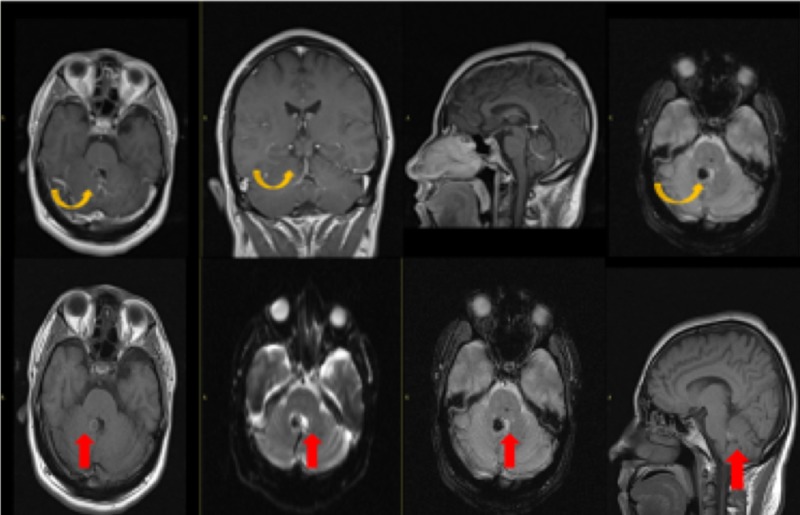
Magnetic Resonance Imaging with Contrast Post-contrast images demonstrate a focal area of blooming at the previously seen site of high density on CT at the right medial margin of the fourth ventricle without enhancement on post-contrast (yellow arrow) representing cavernoma. There is also demonstration of caput medusae appearance of small branching veins draining into a single vein adjacent to this lesion (red arrow) suggestive of a developmental venous anomaly (DVA).
